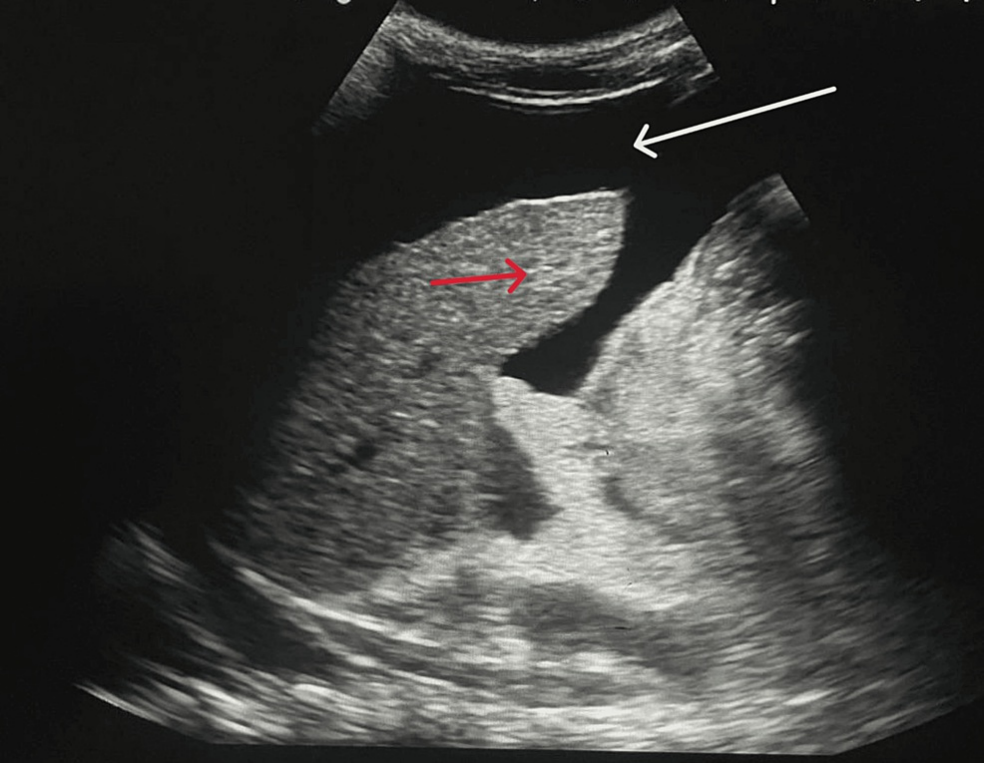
Ultrasound of the abdomen of the patient suggestive of hepatomegaly with raised echotexture of the liver (red arrow) showing grade II fatty liver and moderate ascites (white arrow)
